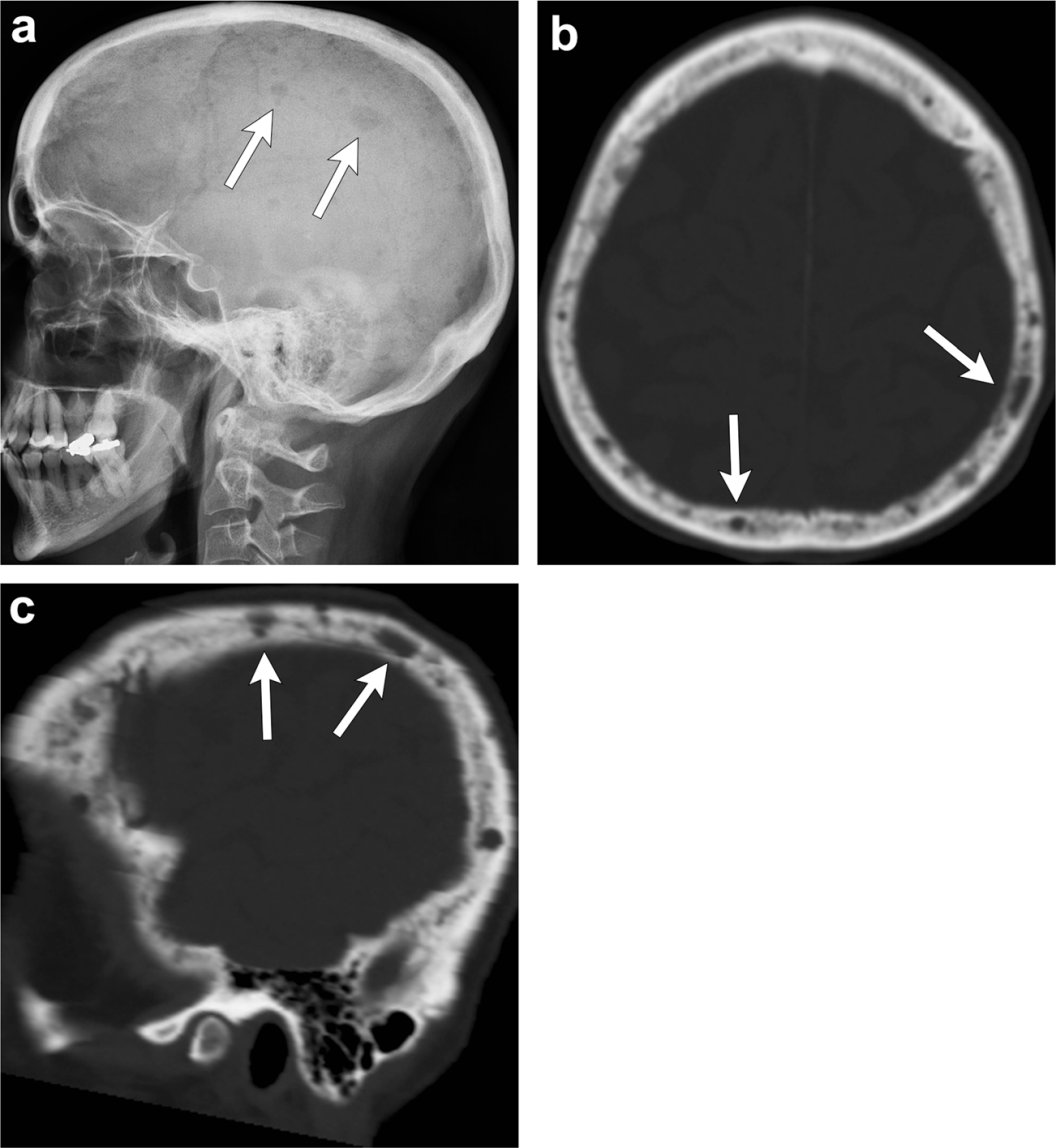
Multiple myeloma. Skull radiograph (a), axial (b) and sagittal (c) head CT show multiple “punched out” lytic lesions in the calvarium (*arrows*), “salt and pepper” appearance
